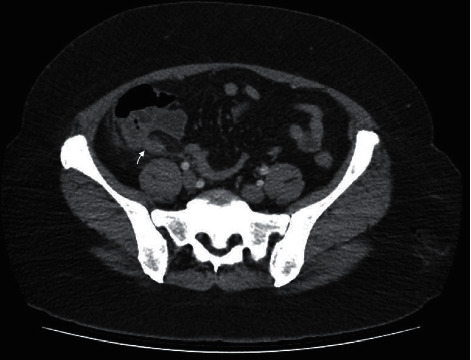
CT findings consistent with the diagnosis of acute appendicitis are observed.
